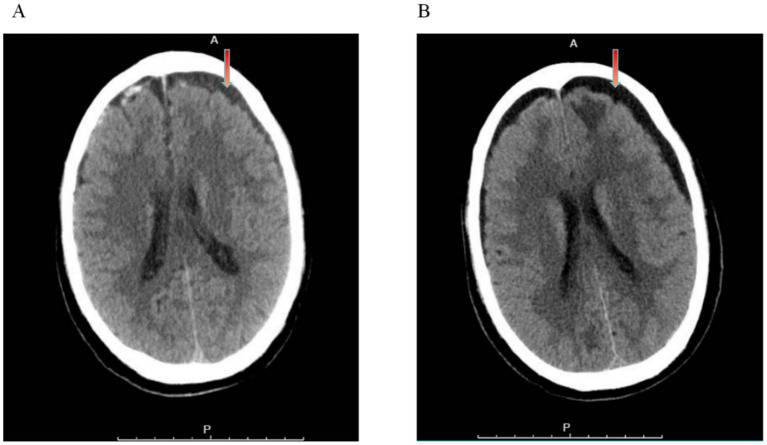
Serial non-contrast computed tomography (NCCT) of the brain. (A) Admission NCCT scans. Axial images demonstrate bilateral frontal hypodense fluid collections with hyperdense sediment levels (red arrows). These findings are consistent with pre-existing chronic subdural hygromas with acute traumatic hemorrhage, a common phenomenon in the elderly. Acute cerebral contusions are also visible in the bilateral frontal and temporal regions. (B) Follow-up NCCT on hospital day 8 (during neurological decline). Axial image at a comparable level shows stable post-traumatic changes with resolving hemorrhage. There is no evidence of new mass effect, midline shift, or herniation to explain the acute deterioration in consciousness.
